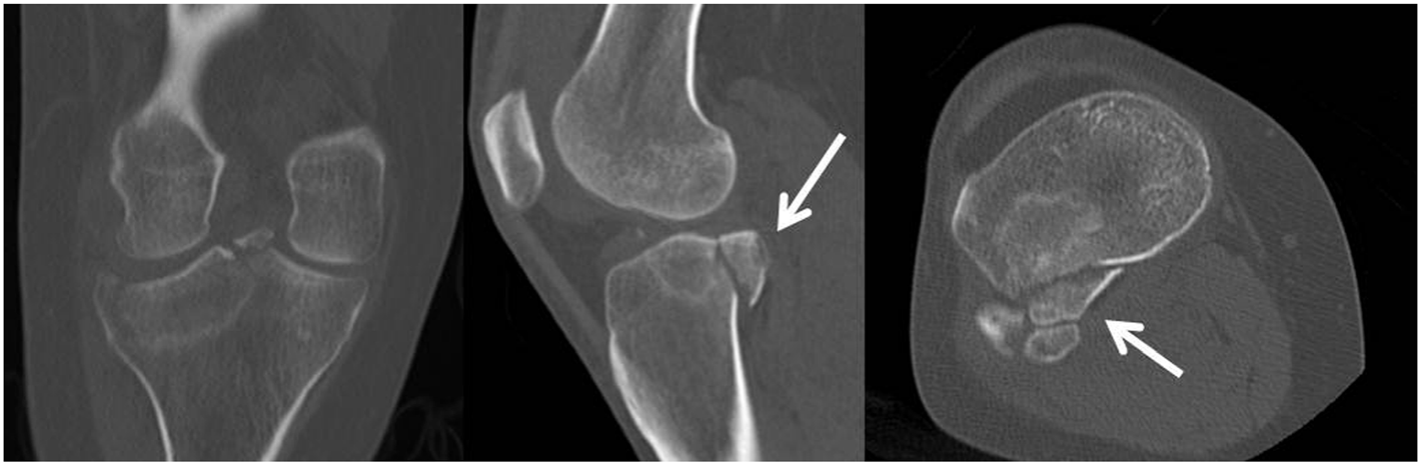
Based on the adverse experience depicted in Figure 4, a breach of the posterior wall and presence of a postero-lateral split fragment (arrows) is considered as a contraindication for the use of inflatable bone tamps. Preoperative radiographs and CT scans must be scrutinized accordingly.
